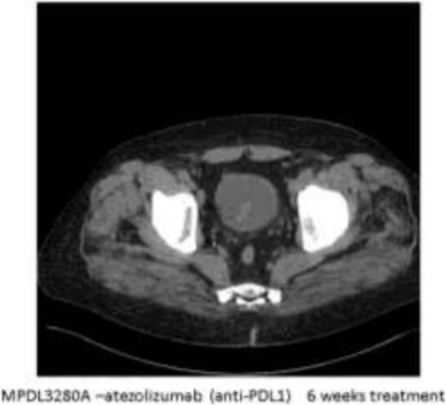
Bladder CT - 9 Weeks of Therapy. 83% Reduction
